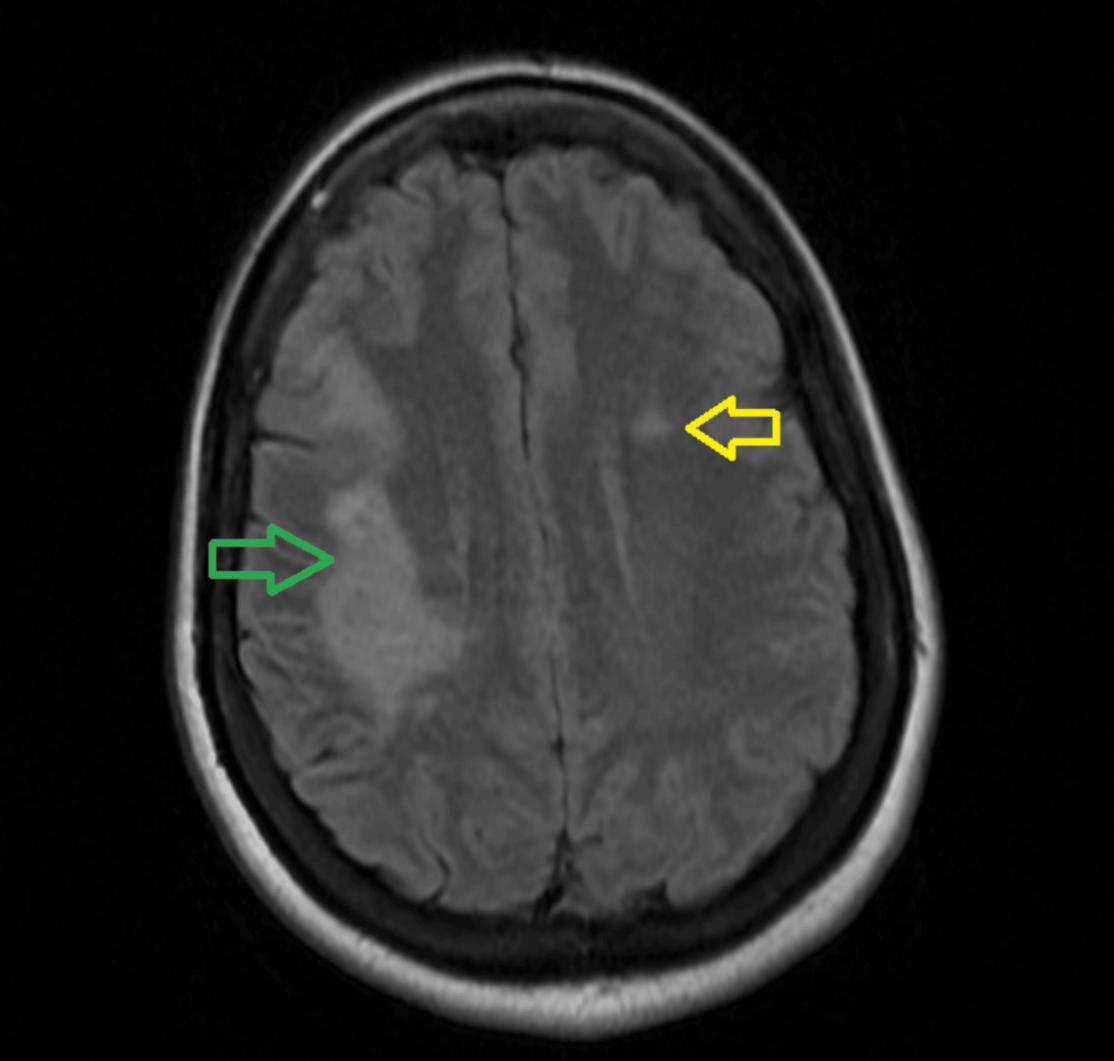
Initial MRI brain with and without contrast - Axial T2 FLAIR FLAIR:  Fluid attenuated inversion recovery. The green arrow indicates a moderate area of hyperintensity within the subcortical, periventricular, and deep white matter of the right frontal and parietal lobes. The yellow arrow indicates a  small, nonspecific foci of increased T2/FLAIR signal in the left frontal and periventricular white matter
